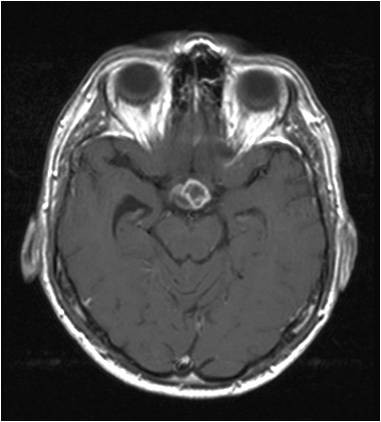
Axial brain MRI of a 51 year old male.
